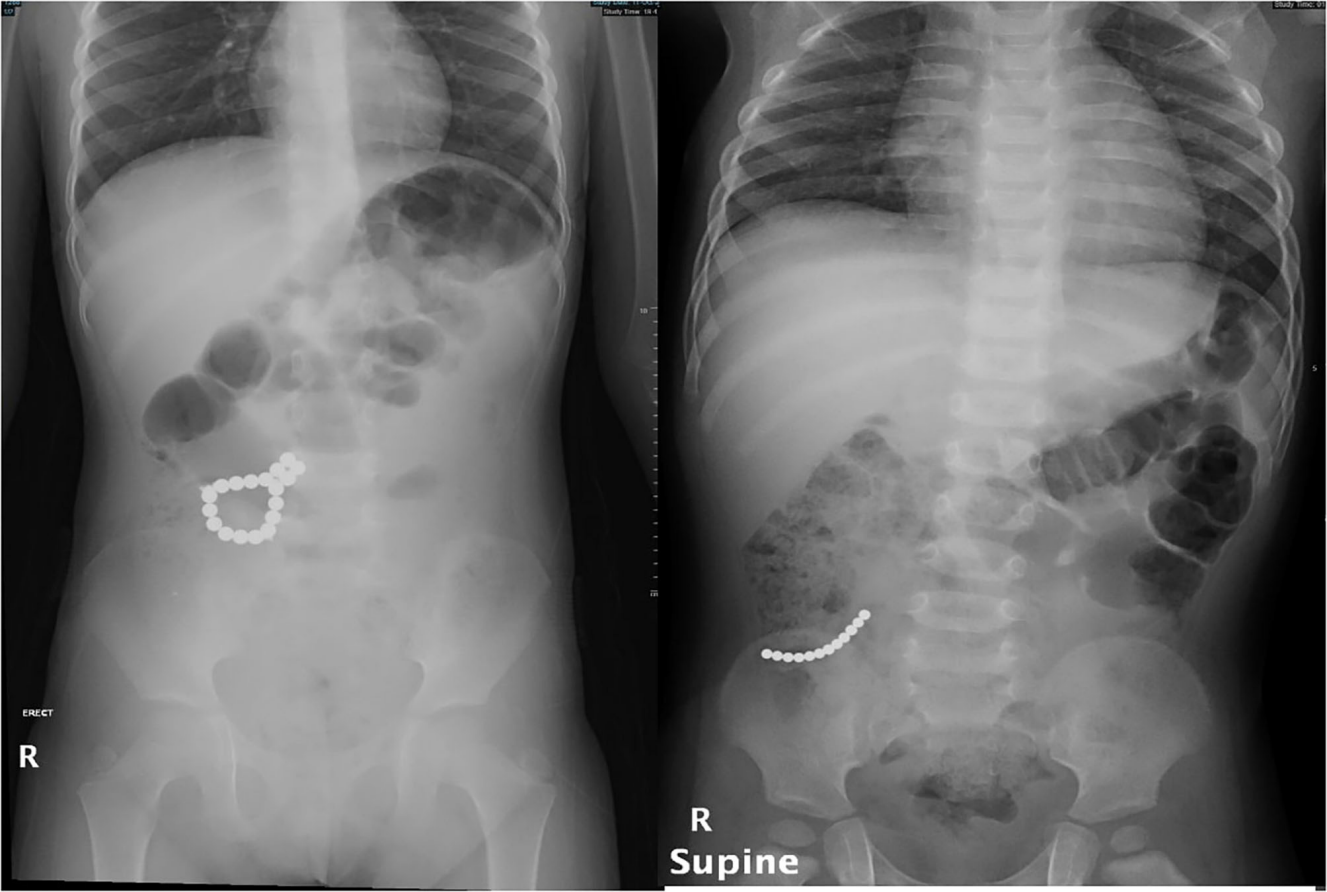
Abdominal radiograph of two patients who presented with non-specific abdominal symptoms.
